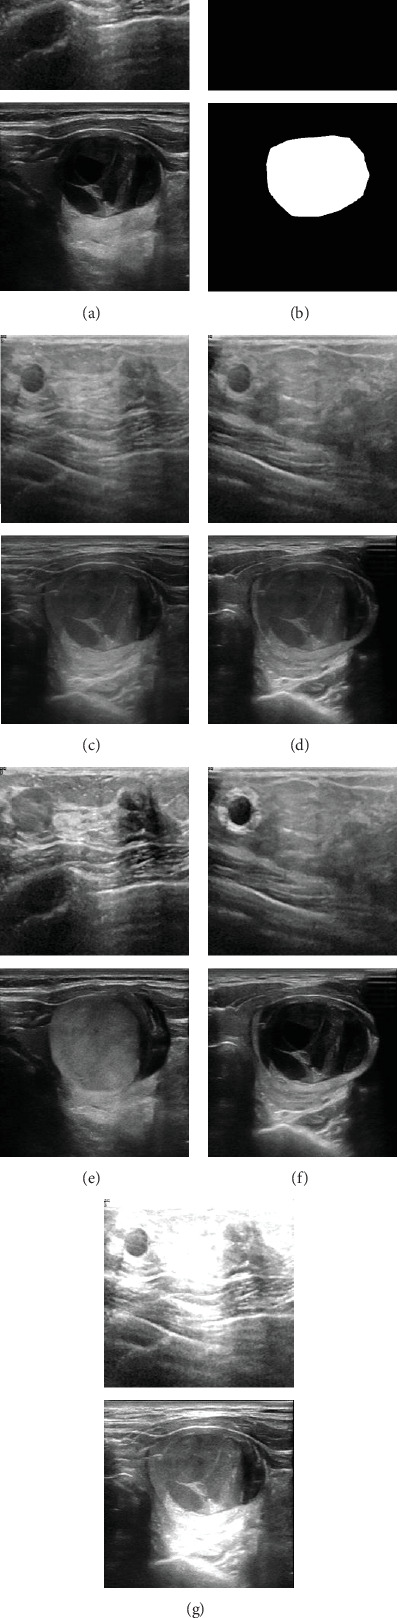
Visual comparison between the original images and the augmented samples. The first row presents breast ultrasound images, and the second row presents thyroid ultrasound images. (a, b) The original image and its corresponding lesion mask, respectively. (c–g) The augmented results generated by the PixMix, SegMix2, LesionBlend, LesionBlend2, and PixMix2 methods, respectively.
